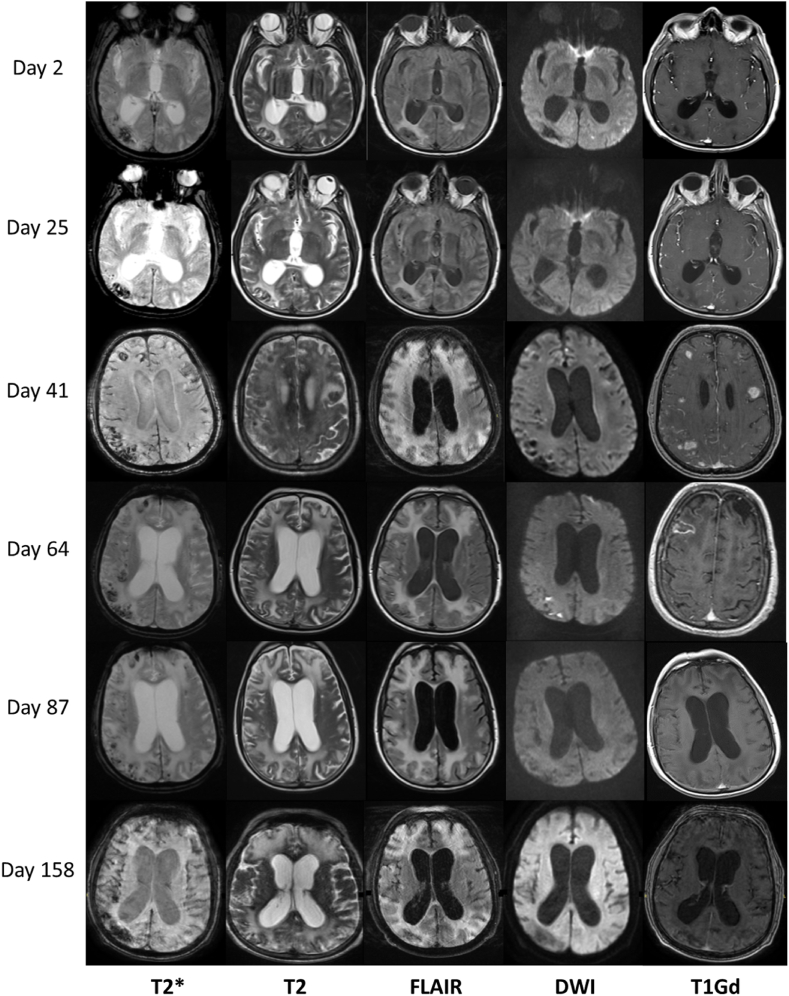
Longitudinal brain imaging findings at certain days post-hospitalization. Left column (T2* sequences): hypointense signal, indicative of hemorrhagic lesions, observed in the right temporooccipital and parietooccipital lobe, as well as left frontal lobe. Second and third columns (T2 and FLAIR sequences respectively): sites of gliosis (hyperintense signal) initially observed in the right parietooccipital lobe, gradually increasing in size and spreading to both cerebral hemispheres (indicative of an leukoencephalopathy), at the level of corona radiata, reaching the maximum exacerbation on the 41st day. Gradual attenuation of the leukoencephalopathy in the subsequent images, although diffuse sites of gliosis remain in the frontal and parietal lobes. There is a gradual increase in the volume of the ventricular system, in the context of brain atrophy. Fourth column (DWI sequences): no restriction in water diffusion is observed but two small focal sites in the right parietal lobe on the 64th day most likely of hemorrhagic origin. Last column (gadolinium enhanced T1 sequences): subacute, focal hemorrhagic sites, giving an increased signal intensity in the frontal and parietal lobes bilaterally, and in the right parietooccipital lobe on the 41st day, combined with right-sided leptomeningeal enhancement. Pathologically increased signal intensity in an area of the right frontal lobe on the 64th day (most likely due to brain biopsy). FLAIR: fluid-attenuated inverse recovery, MRI: magnetic resonance imaging, DWI: diffusion-weighted imaging.
